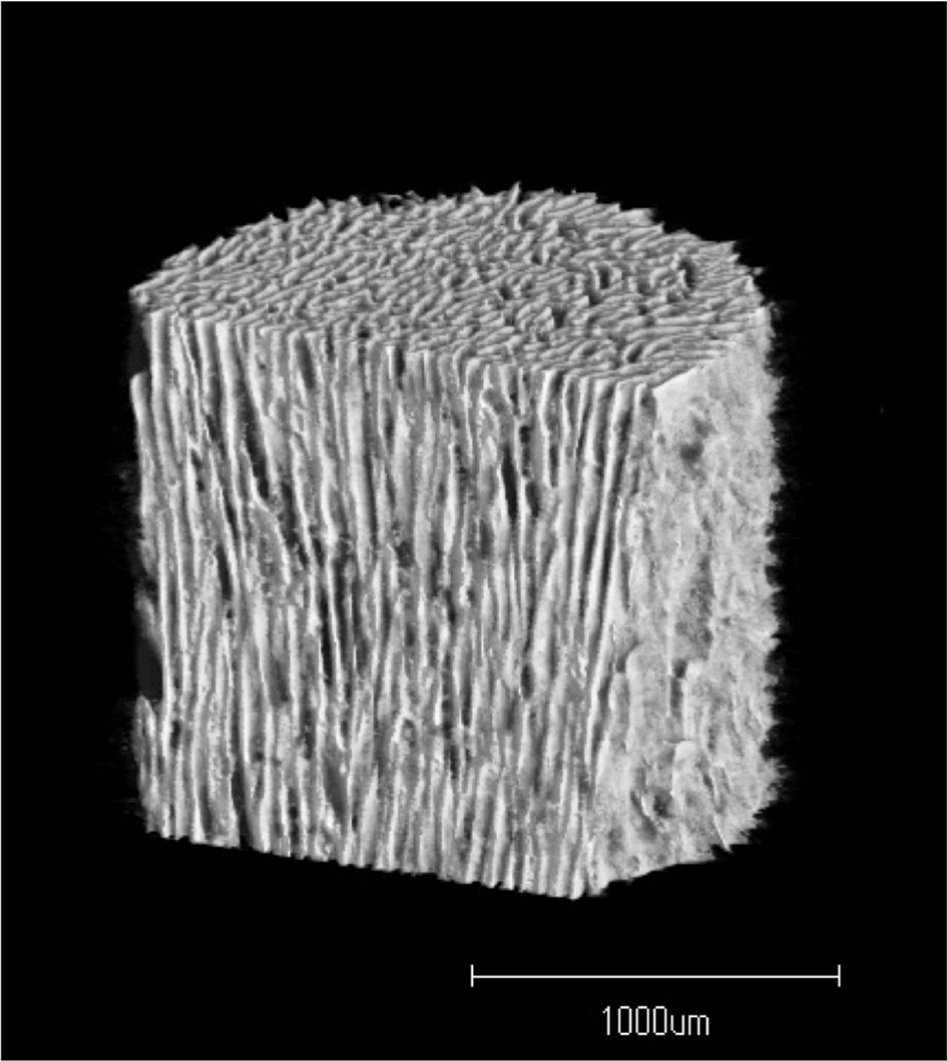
Three-dimensional micro-computed tomography image of unidirectional porous β-tricalcium phosphate (provided by Kuraray) showing unidirectional pores in the vertical direction and some interconnection toward the horizontal direction at the top.
